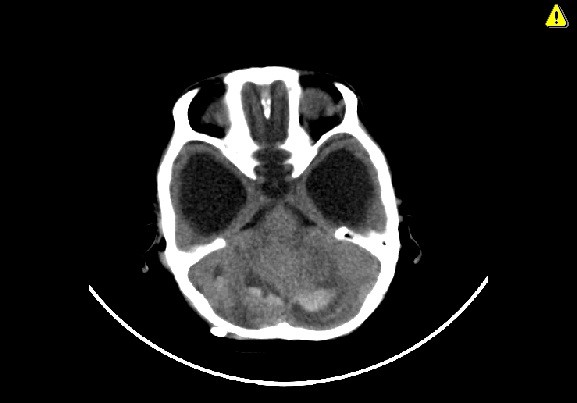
Axial computed tomography scan demonstrating bilateral acute cerebellar hemorrhage.
